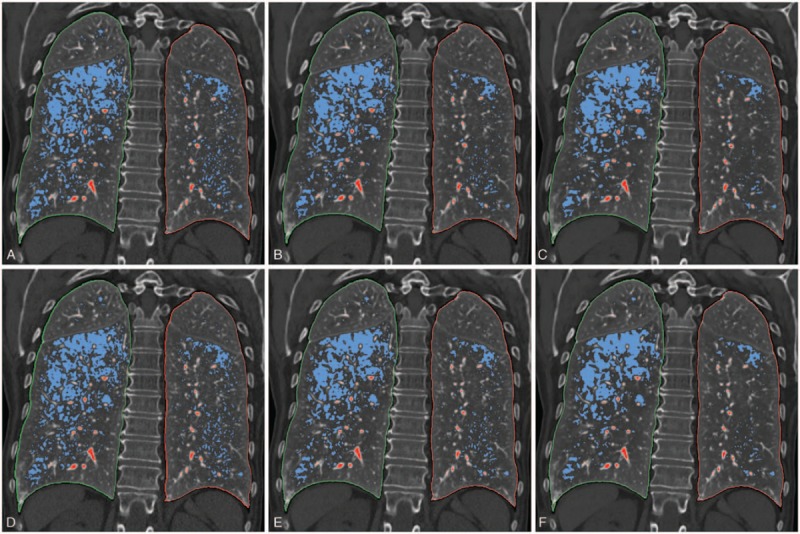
coronal reformatted images of unenhanced chest CT with blue-coloured overlays representing emphysematous lesions from SAFIRE 1 (A), SAFIRE 3 (B), SAFIRE 5 (C), ADMIRE 1 (D), ADMIRE 3 (E) and ADMIRE 5 (F). The lesser confluent blue areas reflect the similar reduction of emphysema index with the increase in the level of IR, best seen in the lower left lobe. ADMIRE = advanced modeled iterative reconstruction, SAFIRE =  sinogram affirmed iterative reconstruction.
